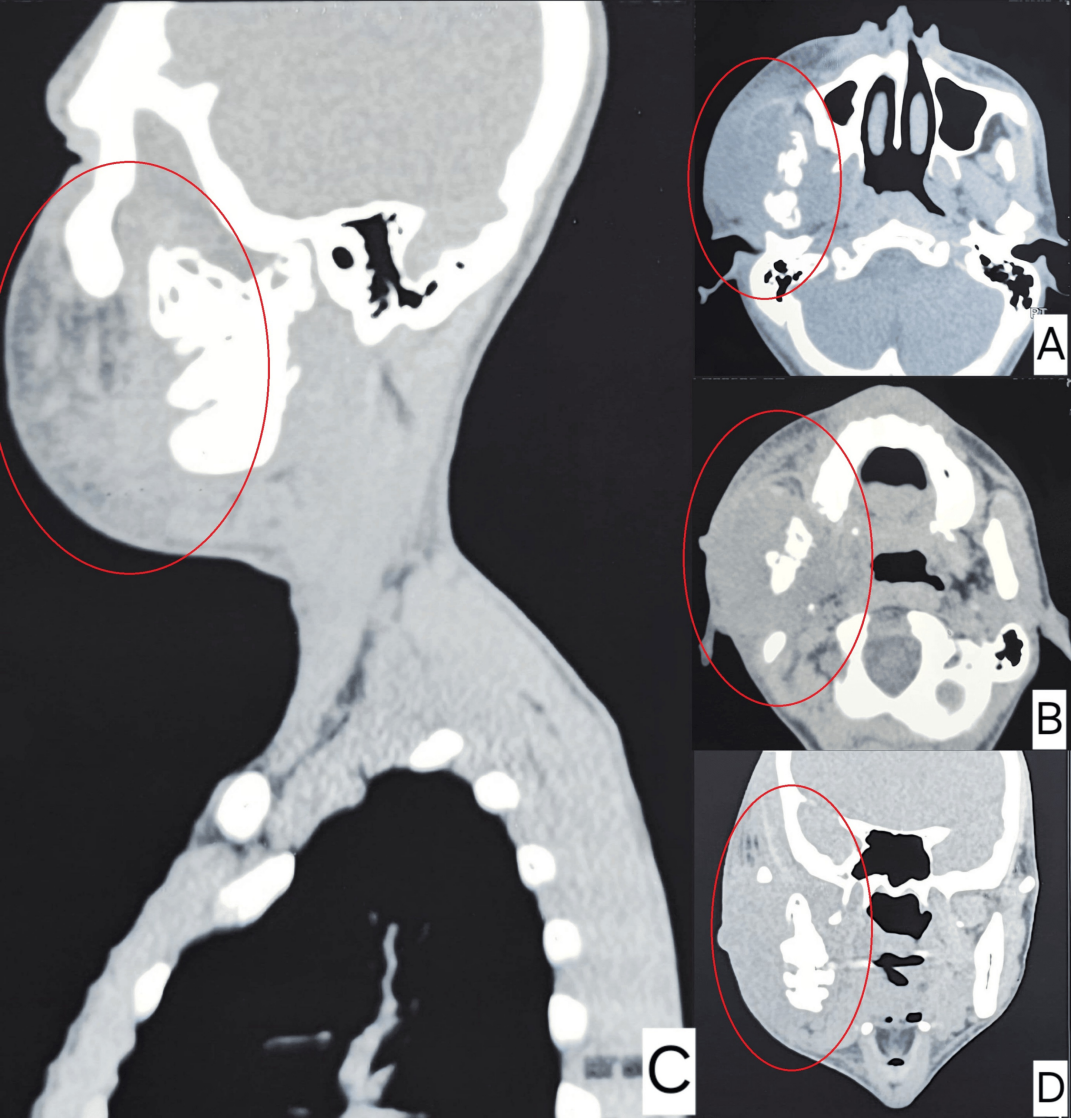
Computed tomography scan image showing heterogeneous soft tissue thickening with bone destruction involving the right ramus of the mandible and the right temporomandibular joint. Irregularly marginated heterogeneous soft tissue dense lesion noted around the ramus of the right hemimandible causing lysis with increased bone marrow attenuation involving the same. Few ill-defined hypodense areas were noted within, likely representing necrosis/evolving abscess. No calcification was noted (B, C, and D). Laterally, the soft tissue-dense lesion involves the master muscle reaching the skin surface. No definite focal defect in the skin surface suggesting fistula formation (B and D). Medially, involving both medial and lateral pterygoid muscles (B and C). Superiorly, extending into the infratemporal fossa with involvement of the infrazygomatic part of the right temporalis muscle and effacement of the retro maxillary fat pad (B). No significant cervical lymphadenopathy.
